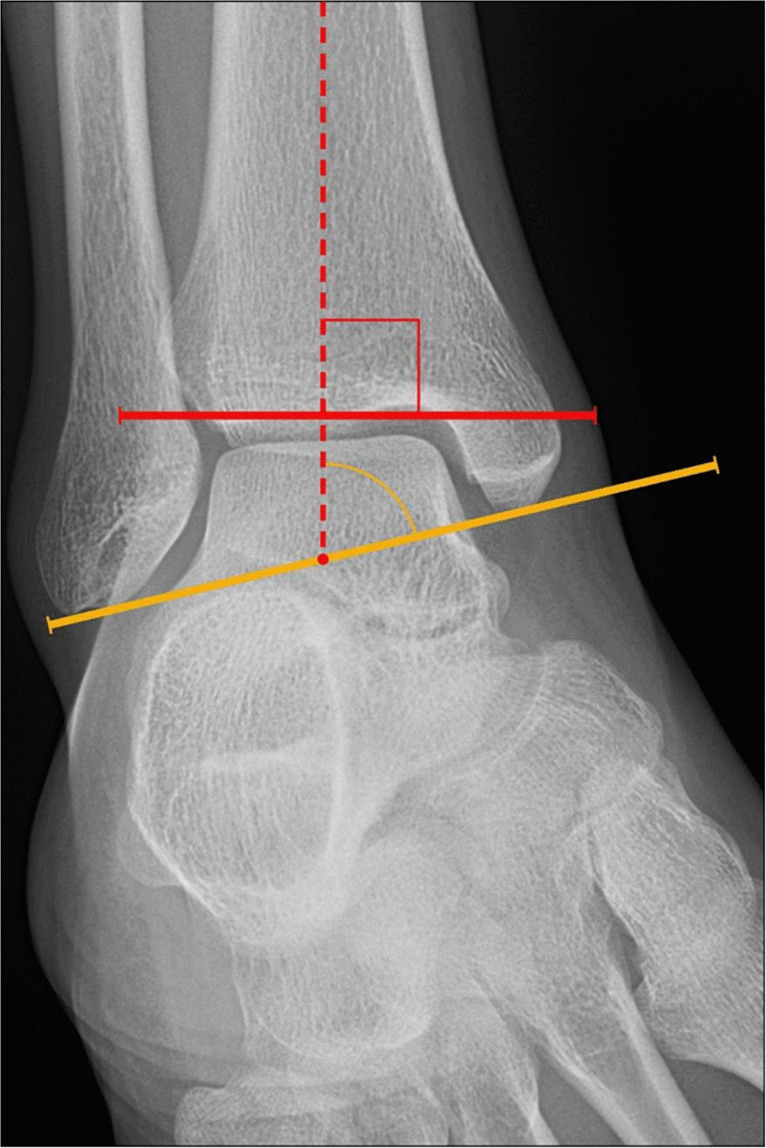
Measurement of the talocrural angle on ankle mortise radiograph. The angle (yellow angle notation) is created from a line connecting the tips of the medial and lateral malleoli (yellow line) and the tibial axis (dashed line), which is perpendicular to the tibial plafond articular surface (solid red line). Comparison with the contralateral side if unaffected may help detect subtle fibular shortening. Normal values are 83° ± 4° with < 2°–4° difference with the contralateral side. When using the tibial axis for the frame of reference, increased angle measurements indicate tibial shortening. Alternatively, the angle between a line tangent to the tibial plafond (solid red line) and a line connecting the tips of the medial and lateral malleoli (solid yellow line) may be taken, with normal values of 8°–15°. When using the tibial plafond as the reference line, decreased angles suggest lateral malleolar shortening
